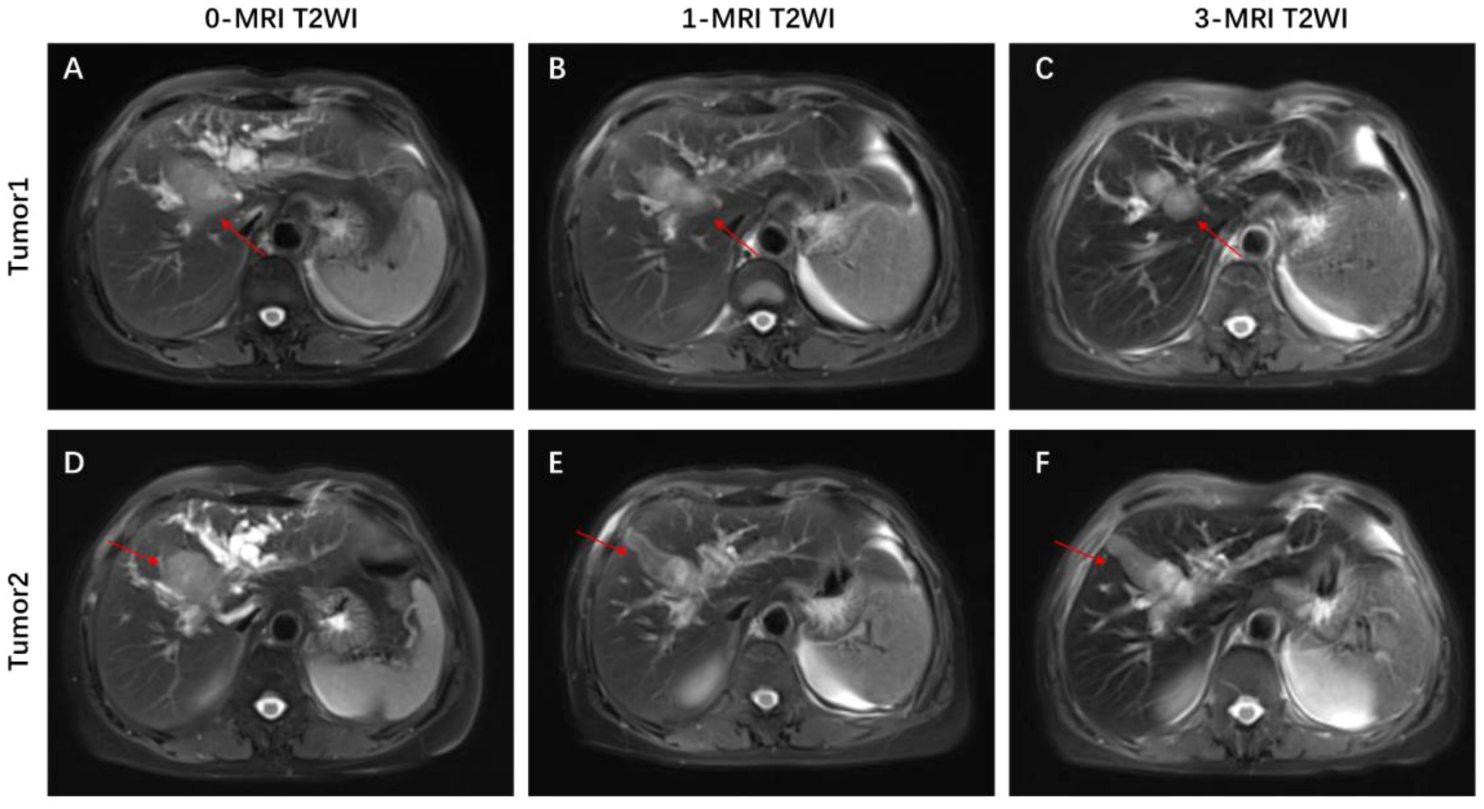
Representative MRI images showing changes in the tumor before and after combination therapy. (A, D) axial T2-weighted MRI images of the liver before combination therapy, the tumor consists of two parts. (B, E) Axial T2-weighted MRI image of the liver at 1 cycle of combination therapy, with a shrinkage of the mass near the hilar region and a change in the morphology of the other mass. (C, F) Axial T2-weighted MIR image of the liver at 3 cycles of combined treatment, with significant shrinkage of the mass near the hilar region.
